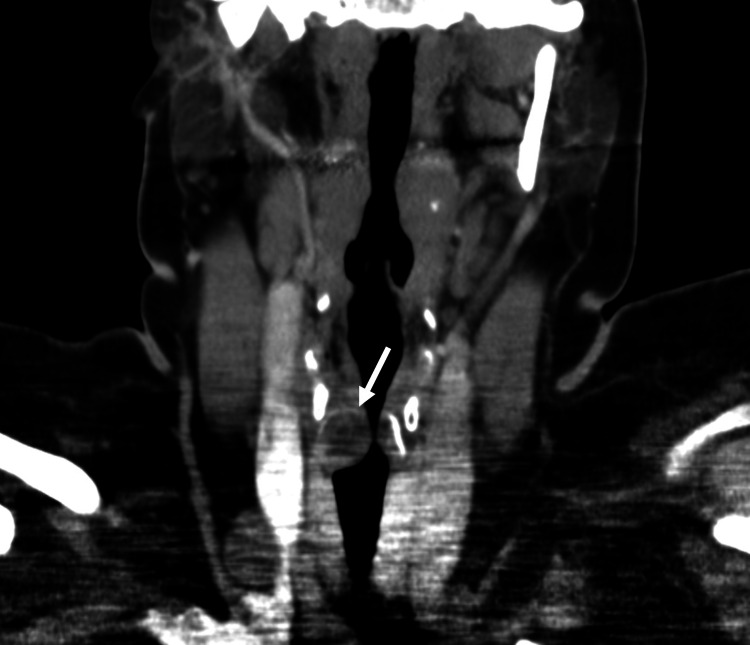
CT scan showing right-sided subglottic mass (2.2 cm x 1.5 cm) (white arrow).
